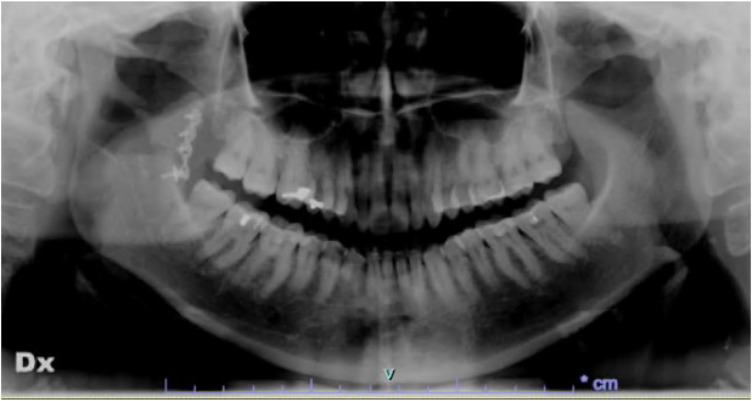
Postoperative orthopantomography (1 week after surgery).
